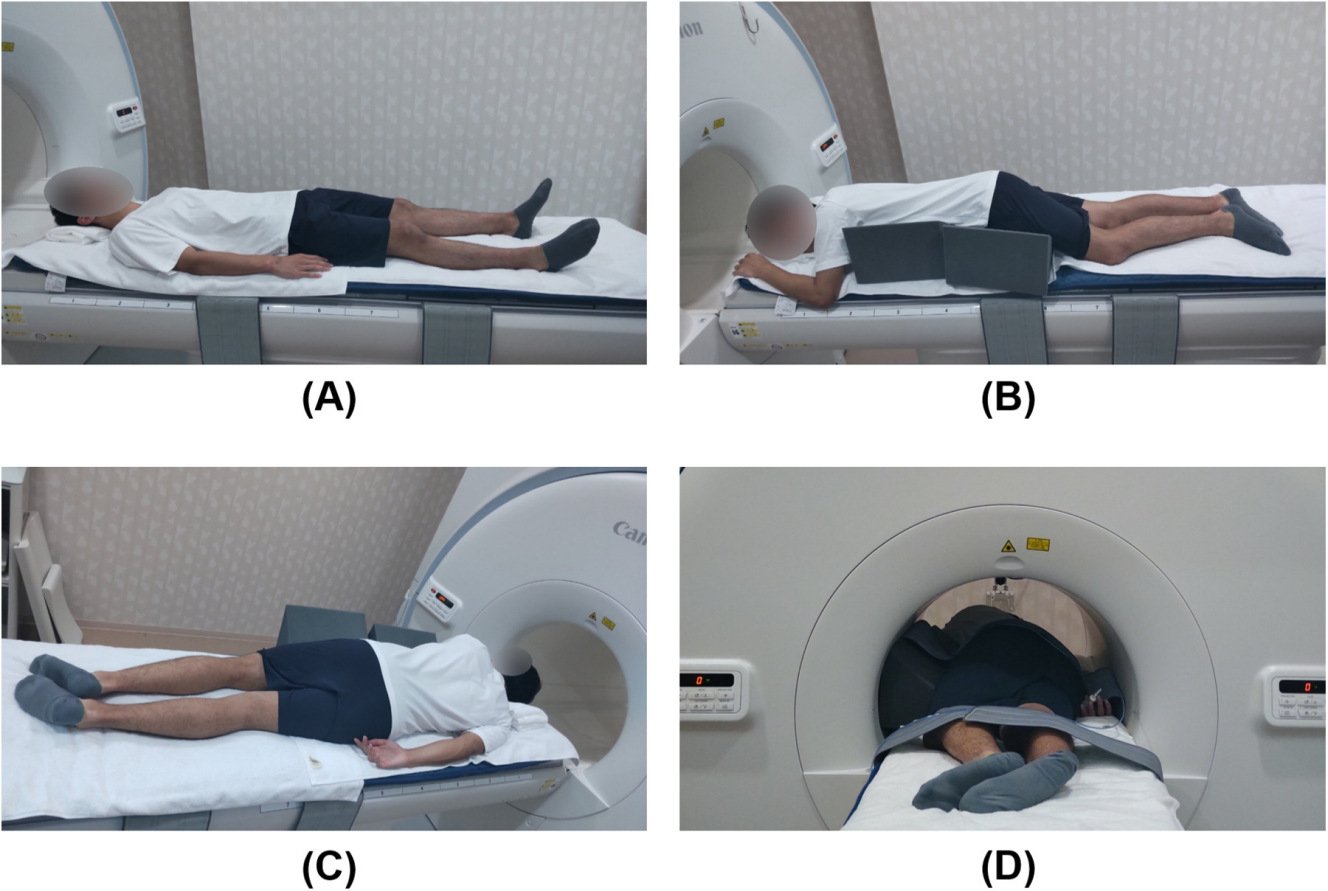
Participant positioning for magnetic resonance imaging. A sleeping mat and towels were placed on the examination table to enhance participants' comfort. (A) Supine position; (B) Semi-prone position with the right side down, supported by a triangular positioning block with an inclination set to 35 degrees to prevent torsion between the pelvic and shoulder girdles; (C) Semi-prone position viewed from the dorsal side; (D) The participant is inserted into the bore in the semi-prone position. There is sufficient space between the participant and the bore, indicating no interference between them.
